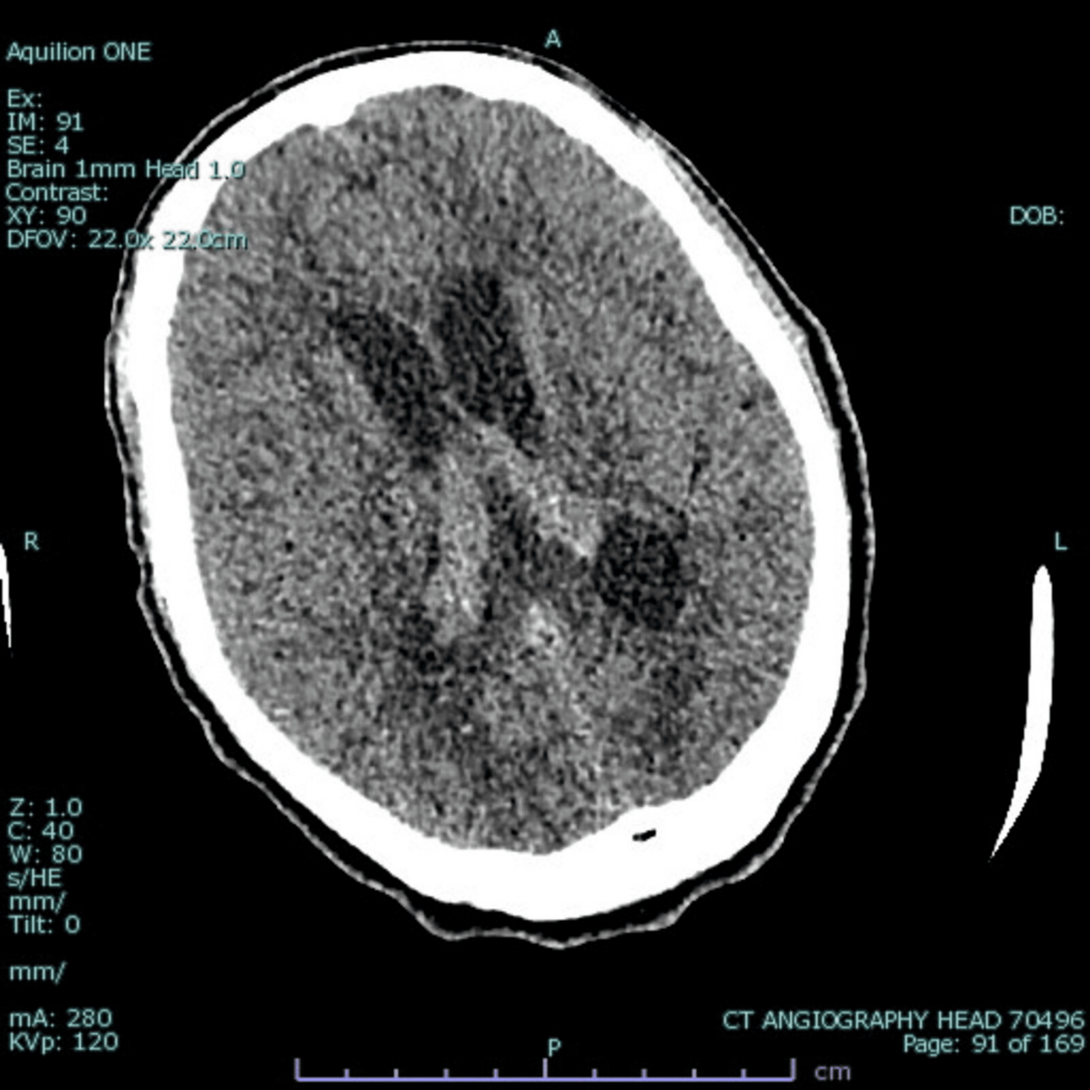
Non-contrast CT of the head showing prominent bilateral choroid plexuses and hydrocephalus affecting the bilateral temporal horns, with surrounding hypodensity
